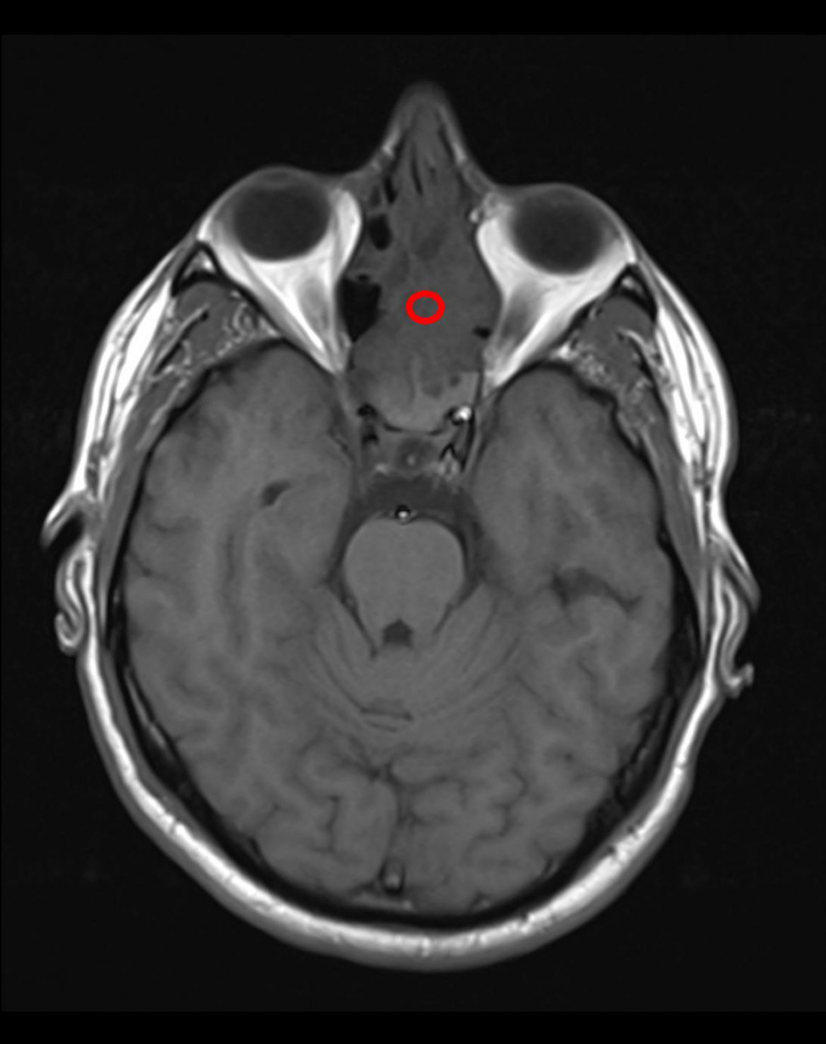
Axial *T*_1_ weighted turbo inversion recovery magnitude MRI reveals a homogeneous isointense mass in the nasal cavity (red circle).
